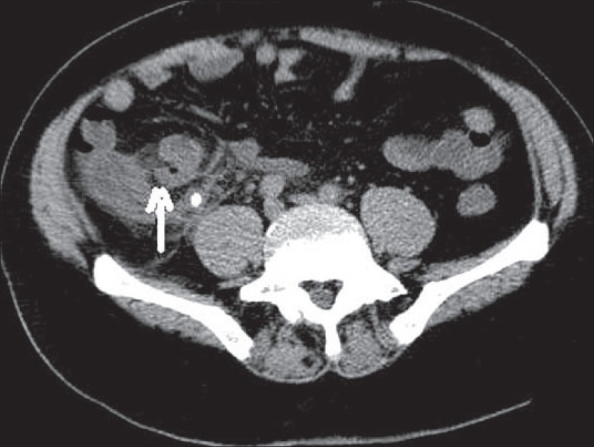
Perforated appendicitis. Plain CT scan shows a dilated appendix with an appendicolith (arrowhead) and periappendiceal abscess formation (arrow)
